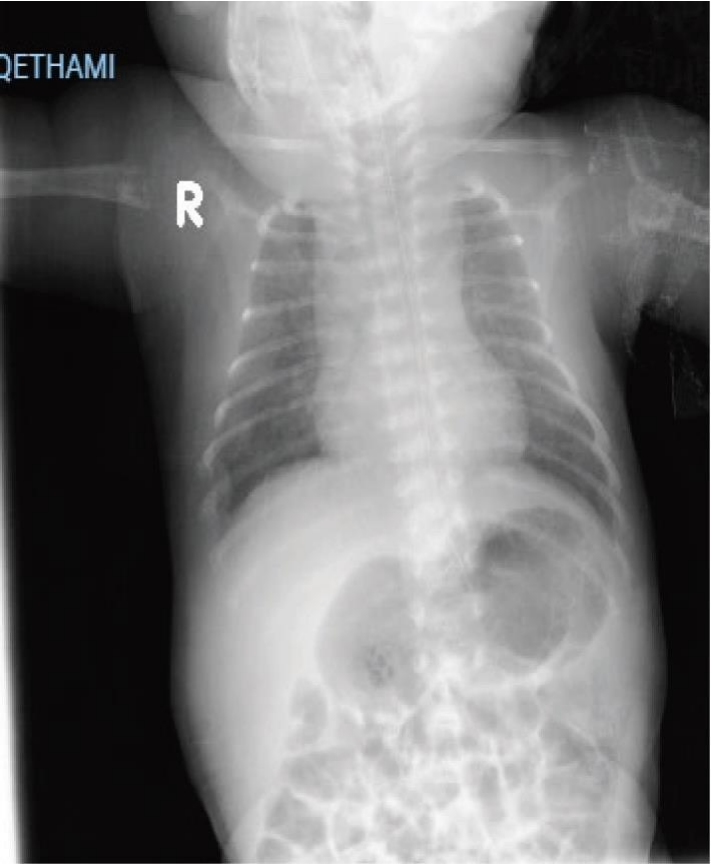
Chest X-ray showing thoracic and pulmonary hypoplasia. Thoracic and pulmonary hypoplasia with thin cortical bone of the ribs.
